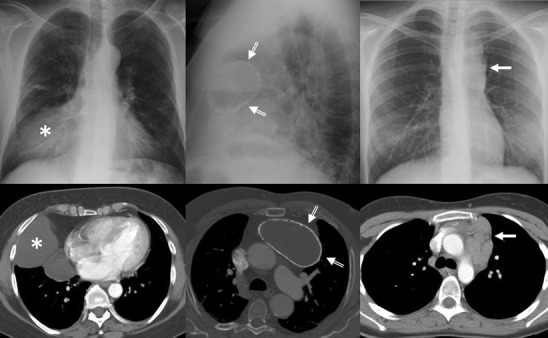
Conventional radiograph can provide information pertaining to the size, anatomical location and density of a central mass. *Right*: Frontal chest radiograph shows a sharply defined area of increased opacity with a loss of the cardiac silhouette at the border of the right side of the heart (*). Contrast-enhanced CT scan reveals a thin-walled water-attenuation lesion (*) in the right cardiophrenic angle (pericardial cyst). *Middle*: Lateral chest radiograph and contrast-enhanced CT scan show a unilocular, well-defined and homogeneously hypodense mass in the anterior mediastinum with peripheral calcification (*open arrows*) (thymic cyst). *Left*: Chest radiograph shows the aortopulmonary window with an abnormal convex border (*arrow*). Contrast-enhanced CT scan demonstrates a multilobulated mass in the anterior mediastinum (*arrow*), which accounts for the distortion of the AP window (nodular sclerosis Hodgkin disease)
